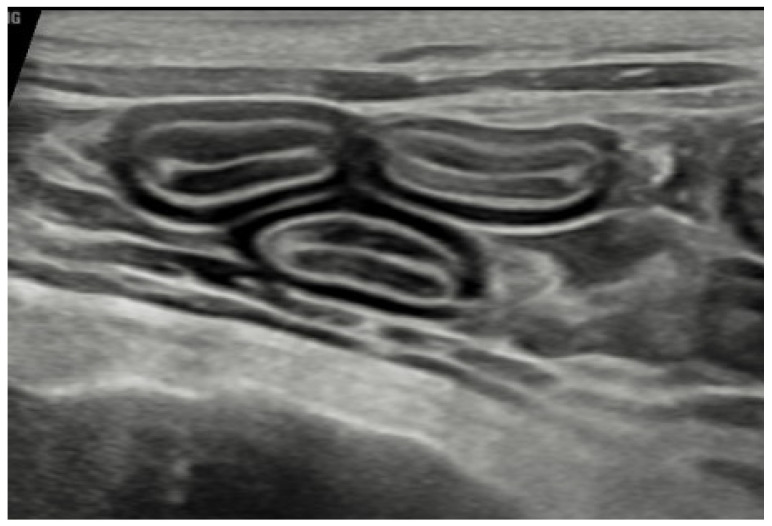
Ultrasound image of loops of jejunum showing mild muscularis thickening.
